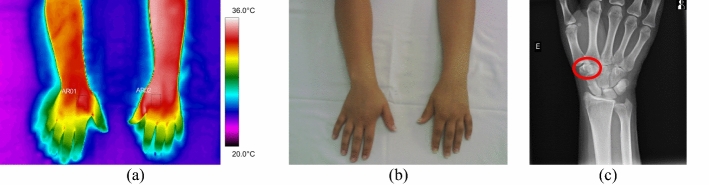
Left scaphoid bone fracture: a infrared thermogram of the volunteer's lower limbs with delimitation of the ROIs; b a photograph for comparative analysis; c corresponding radiography
